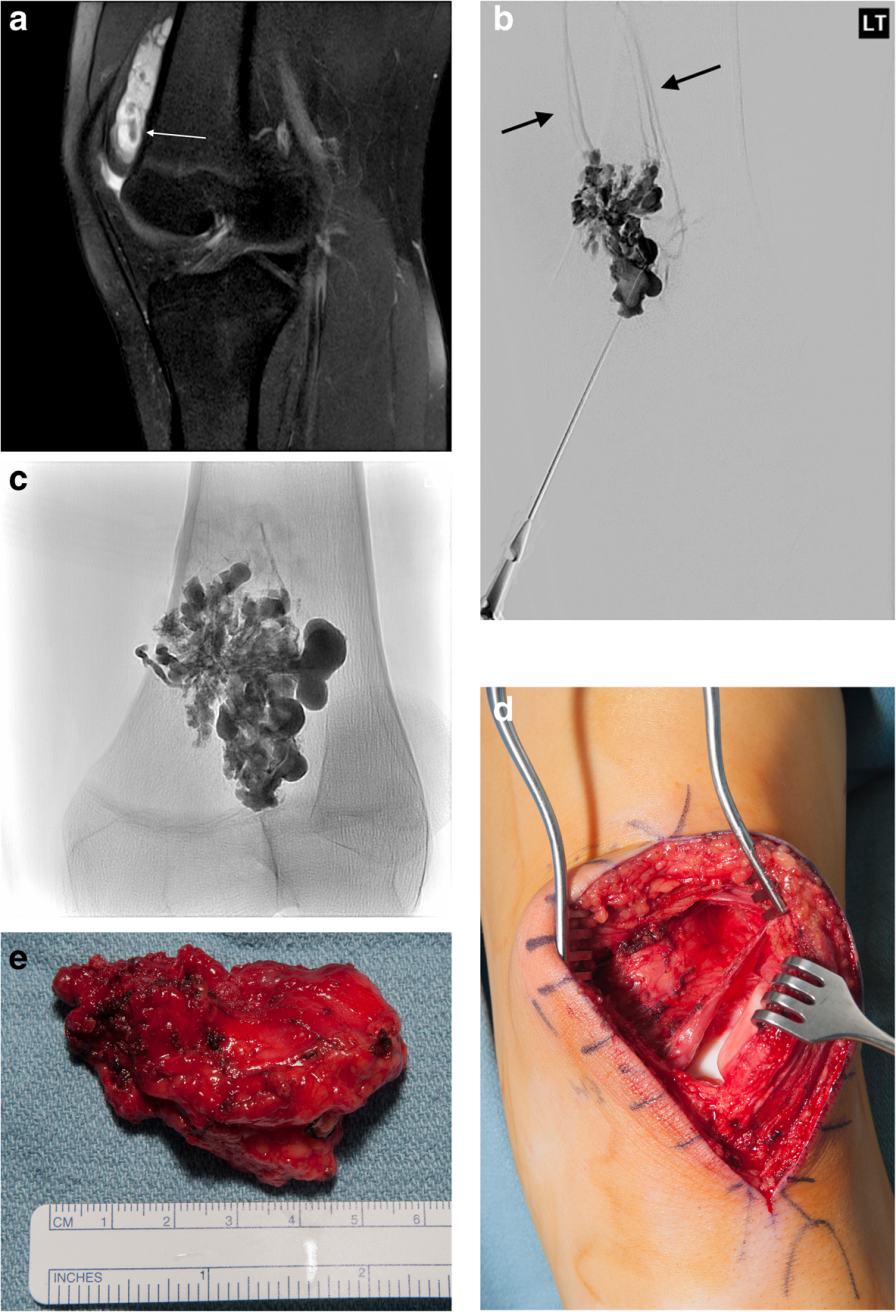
a 14-year-old female with chronic left knee pain and swelling initially misdiagnosed and managed as monoarticular juvenile idiopathic arthritis. Sagittal fat saturated proton density-weighted MRI showing hyperintense channels of the intraarticular venous malformation along with hypointense phleboliths (arrow). The lesion measured 3.4 × 0.9 × 4.5 (SI) cm. b Frontal projection digital subtraction venography after direct percutaneous access of the venous malformation showing opacification of numerous channels with ascending venous outflow (arrows). c
